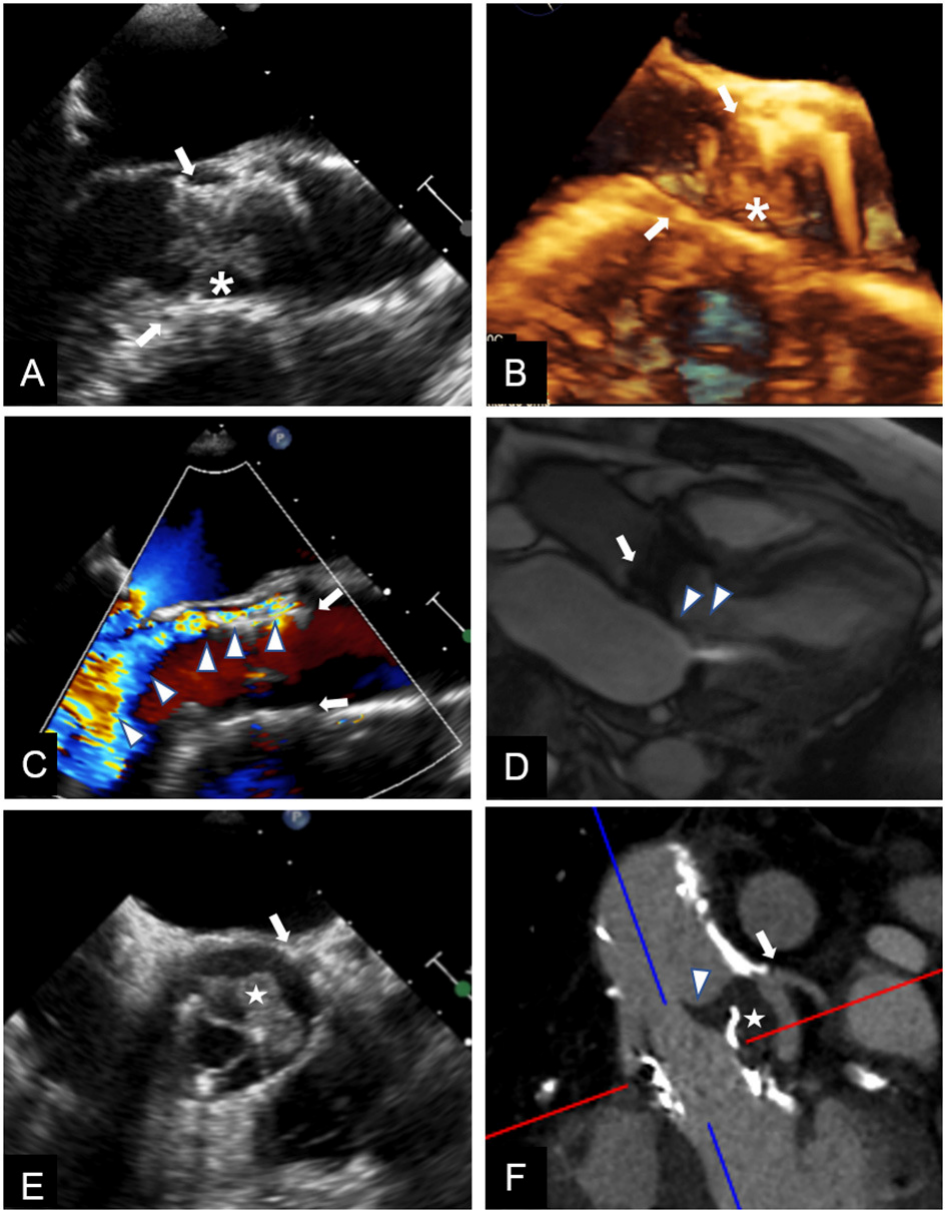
Multimodality imaging for differential diagnosis of high trans-prosthetic gradients in patients with THV. *Case 1*. TEE showing an isoechoic mobile mass (*) adherent to THV (arrows) cusps, causing valve obstruction; blood cultures confirmed infective endocarditis (A,B). *Case 2*. TEE revealing severe paravalvular (arrows) leak (C, arrowheads); the regurgitant jet is also shown during CMR cine sequences (D, arrowheads), and can be quantified using phase contrast sequences. *Case 3*. TEE in a patient known for valve-in-valve procedure showing an isoechoic mass (E, white star) in left coronary sinus, affecting cusp motion (arrowhead). CT (F), confirmed the mass (white star) arising between the two valves, and whose features and density were consistent with thrombus.
